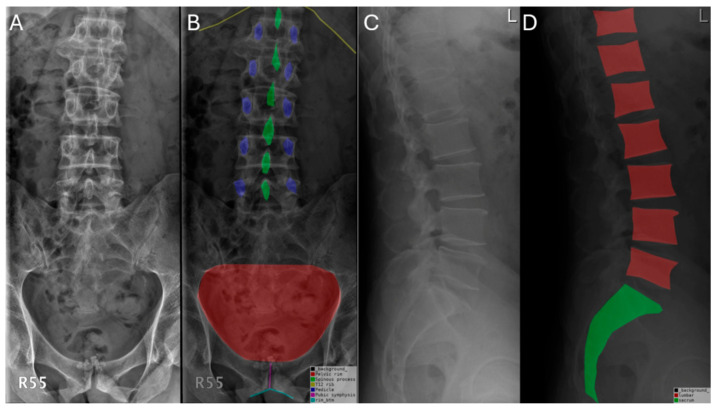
Landmark annotation on anterior–posterior (AP) and lateral (Lat) plain radiographs. (A) Original AP radiograph; (B) AP radiograph with annotated landmarks: spinous process (green), pedicle (blue), T12 rib (yellow), pubic rim (red), pubic symphysis (pink), and inferior margin of the pubic rim (light blue); (C) Original LAT radiograph. (D) LAT radiograph with annotated vertebral bodies from T11 to L5 (red) and sacrum (green).
